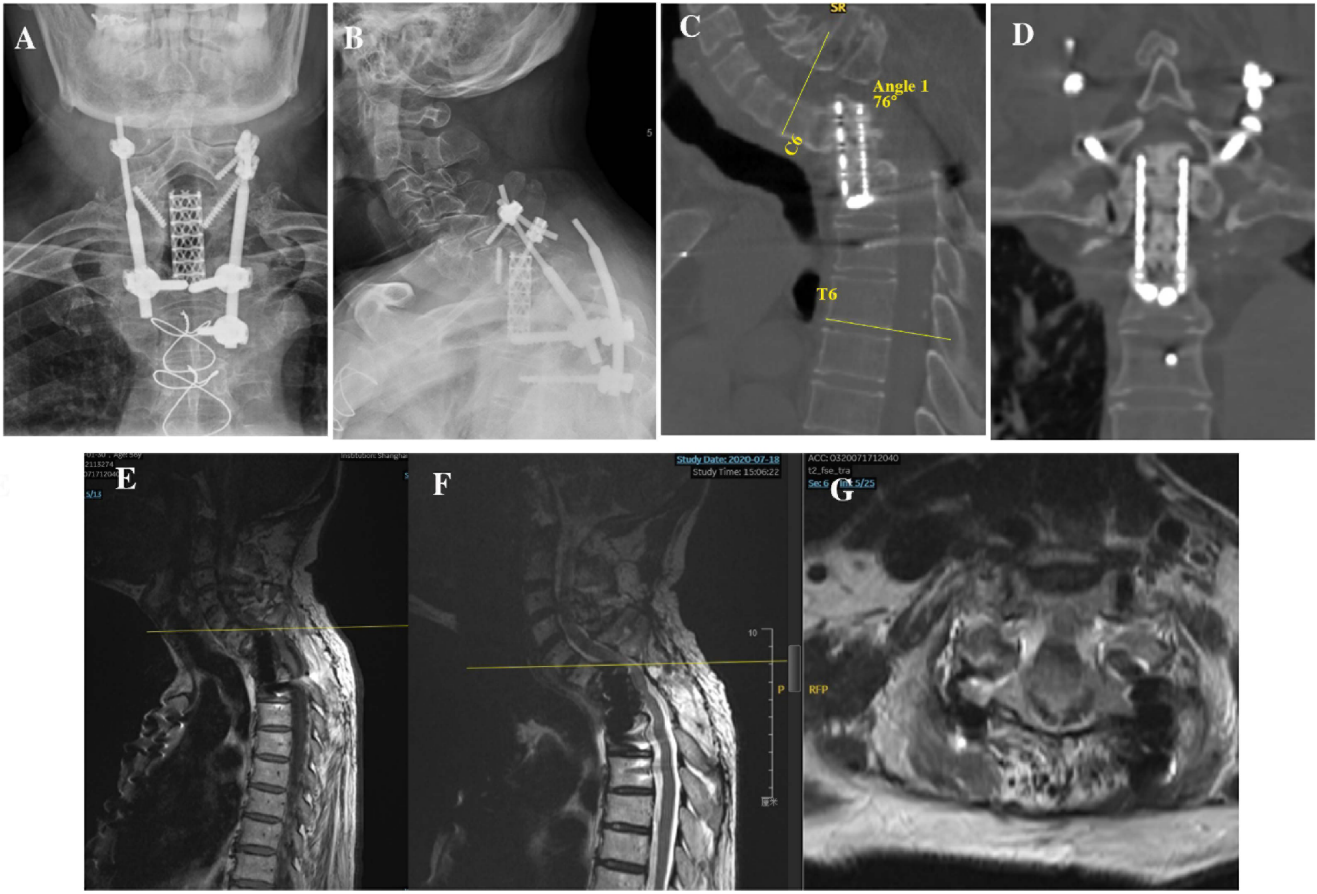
(A,B) Anteroposterior and lateral x-rays 5 year after first surgery showing screw/rod fracture and mesh cage displacement. (C,D) Sagittal and coronal CT showing increased local kyphosis and mesh cage subsidence into the C7 vertebral body. (E–G) T1- and T2-weighted sagittal MRIs and T2-weighted axial MRI images showed compression of spinal cord by subsided mesh cage. CT, computed tomography; MRI, magnetic resonance imaging.
